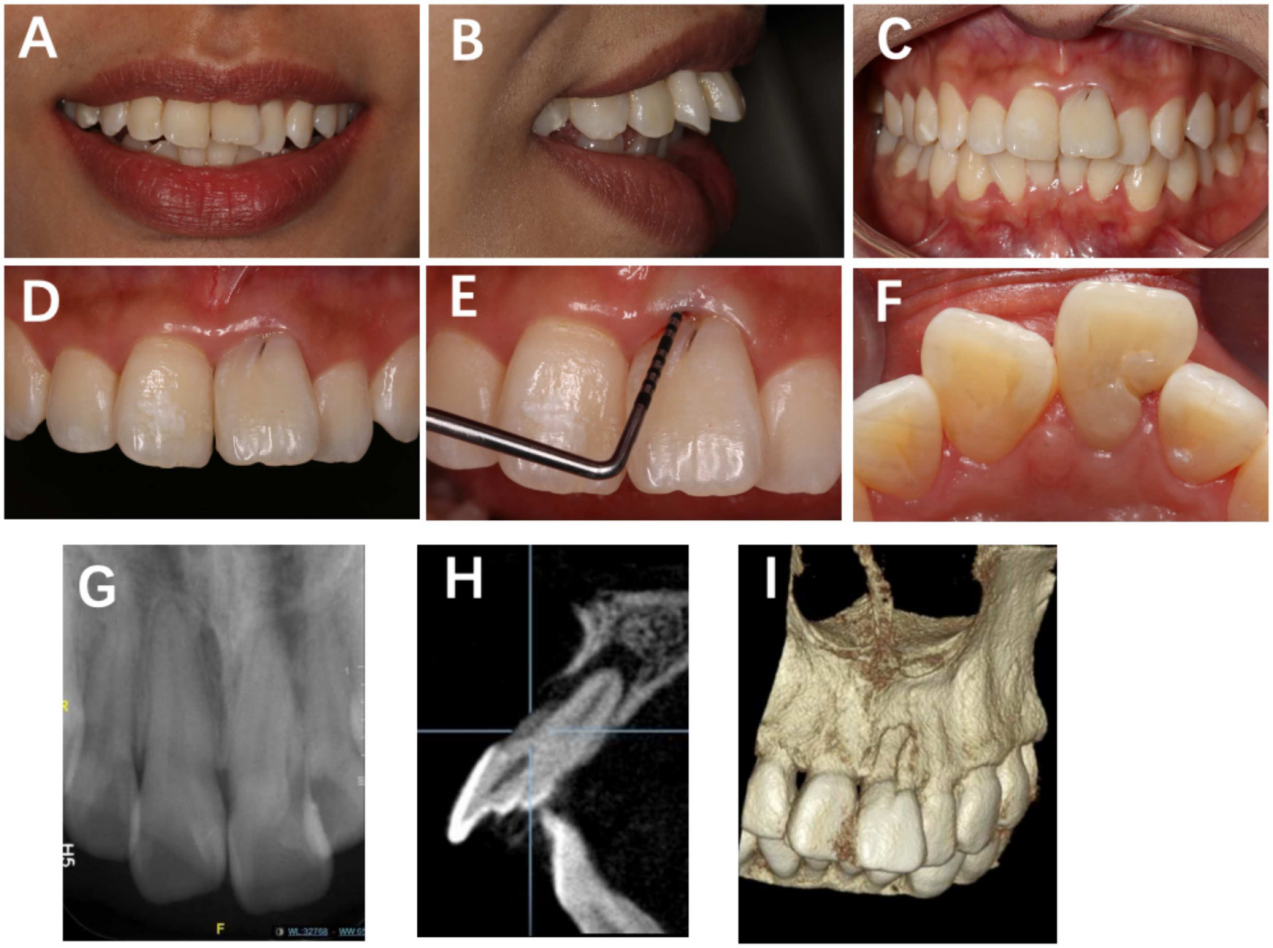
Preoperative clinical view of Tooth #21 showing a distinct labial developmental groove extending from the cingulum toward the gingival margin, accompanied by localized periodontal pocketing. (A) Frontal smile view; (B) lateral smile view; (C) frontal view in centric occlusion; (D) labial surface of Tooth #21 showing a developmental groove; (E) probing depth of 10 mm at the mid-labial site; (F) shallow depression (2 mm) on the distolingual marginal ridge; (G) periapical radiograph showing two root canals, one poorly visualized; (H) periapical radiolucency surrounding the root apex; (I) CBCT 3D reconstruction demonstrating a labial groove along the root surface.
